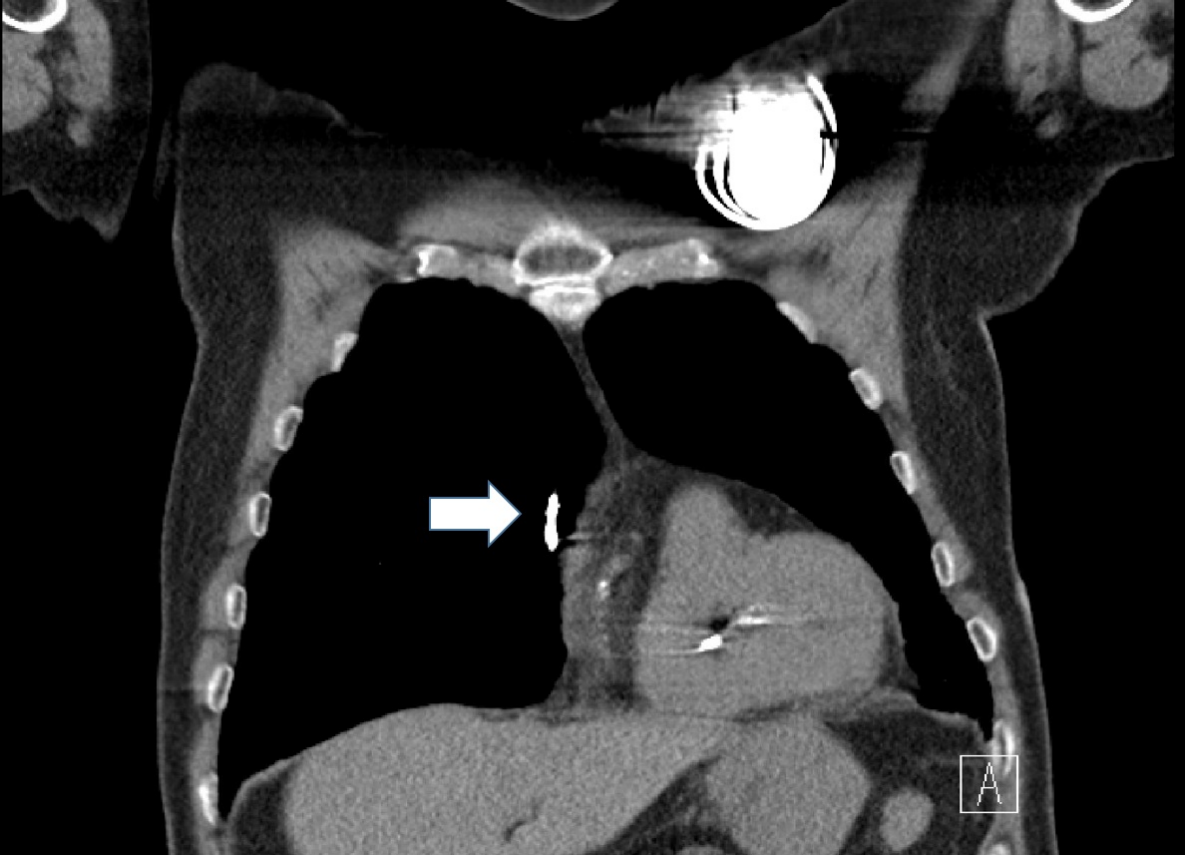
High-resolution computed tomography scan of the chest coronal cut with white arrow pointing at the tip of atrial lead migrating into the right lung
